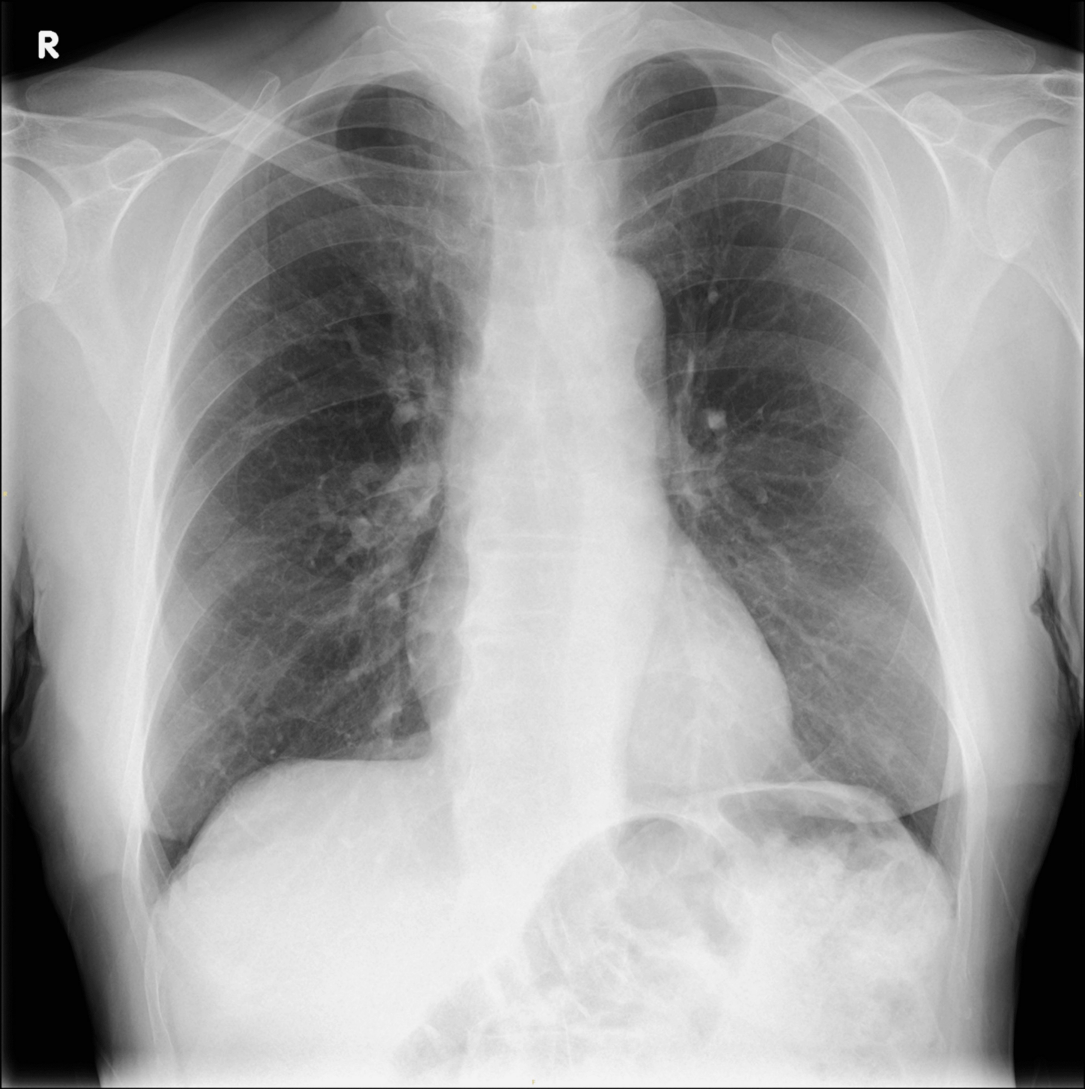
Chest X-ray performed at the three-month follow-up in the respiratory clinic showing complete re-inflation of the left lung.
